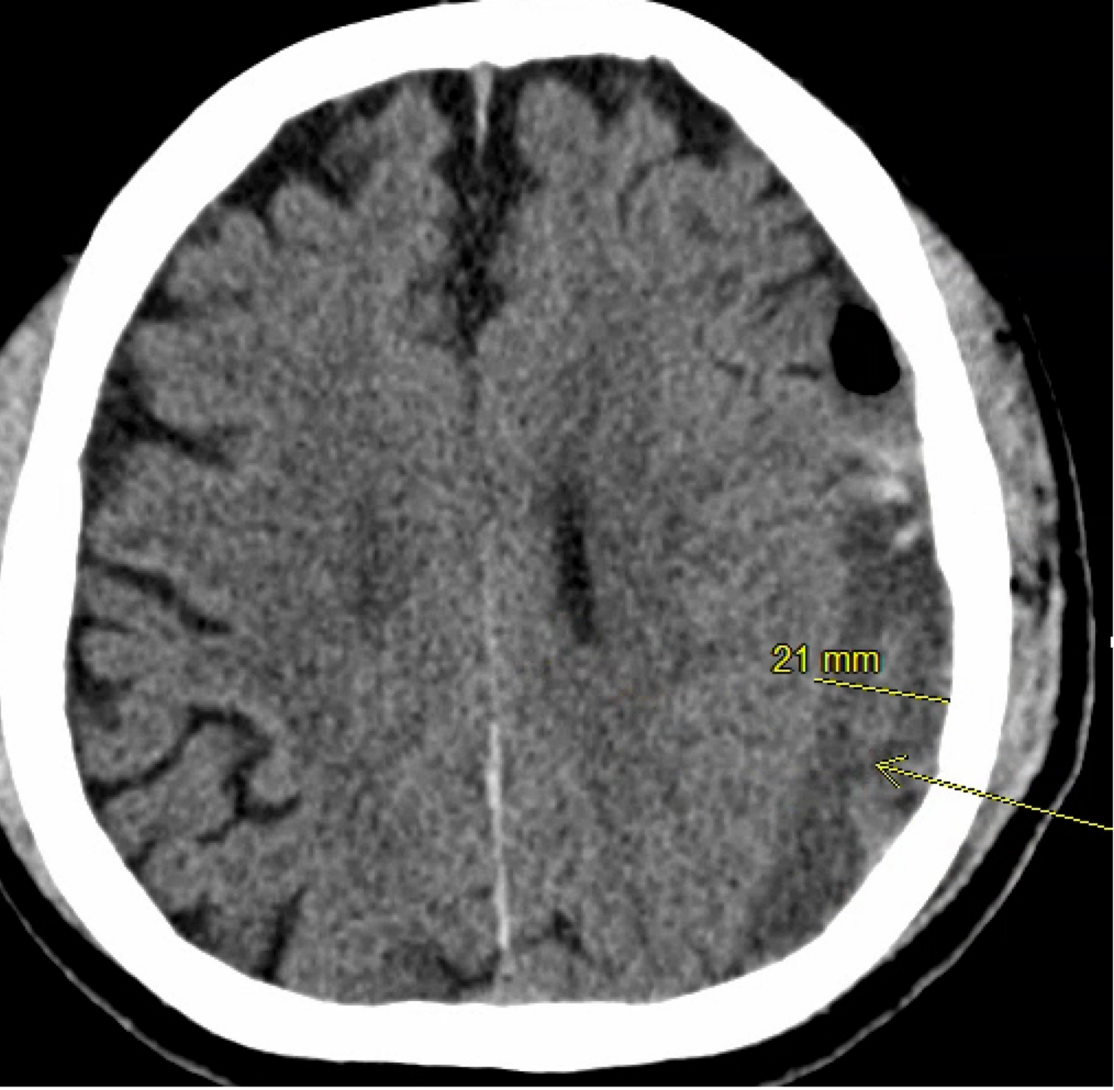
Pre-tissue plasminogen activator CT scan of a patient who underwent subdural drain placement with tissue plasminogen activator adjunct This image was taken prior to the initiating protocol for the administration of tissue plasminogen activator through the subdural drain. Mass effect is decreased but still present from the subdural hematoma, marked by the arrow compared to figure 1. Some pneumocephalus is present from initial drain placement and there is evidence of some post-traumatic hemorrhage caused by drain placement.
